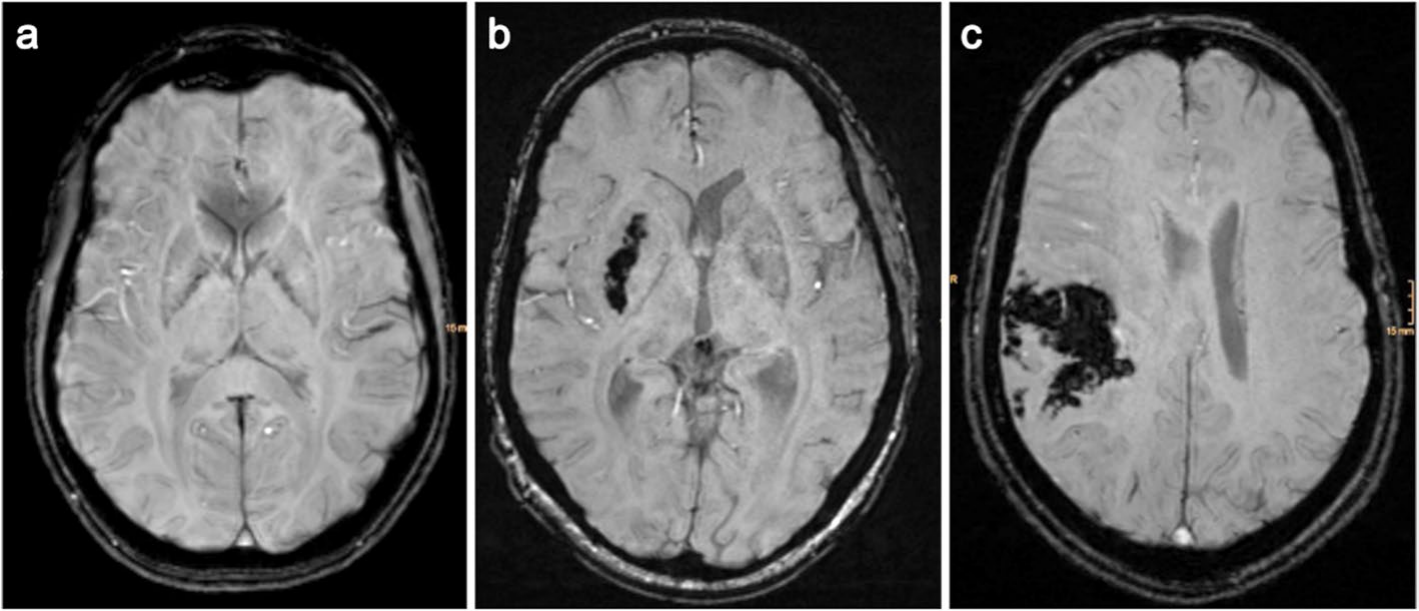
Representative examples of postinterventional 24-h follow-up susceptibility-weighted imaging (SWI) on MRI; hemorrhages classified by The Heidelberg Bleeding Classification: a No hemorrhagic transformation (Heidelberg Class 0). b Hemorrhagic transformation (Heidelberg Class 1). c Significant hemorrhage (Heidelberg Class 2)
